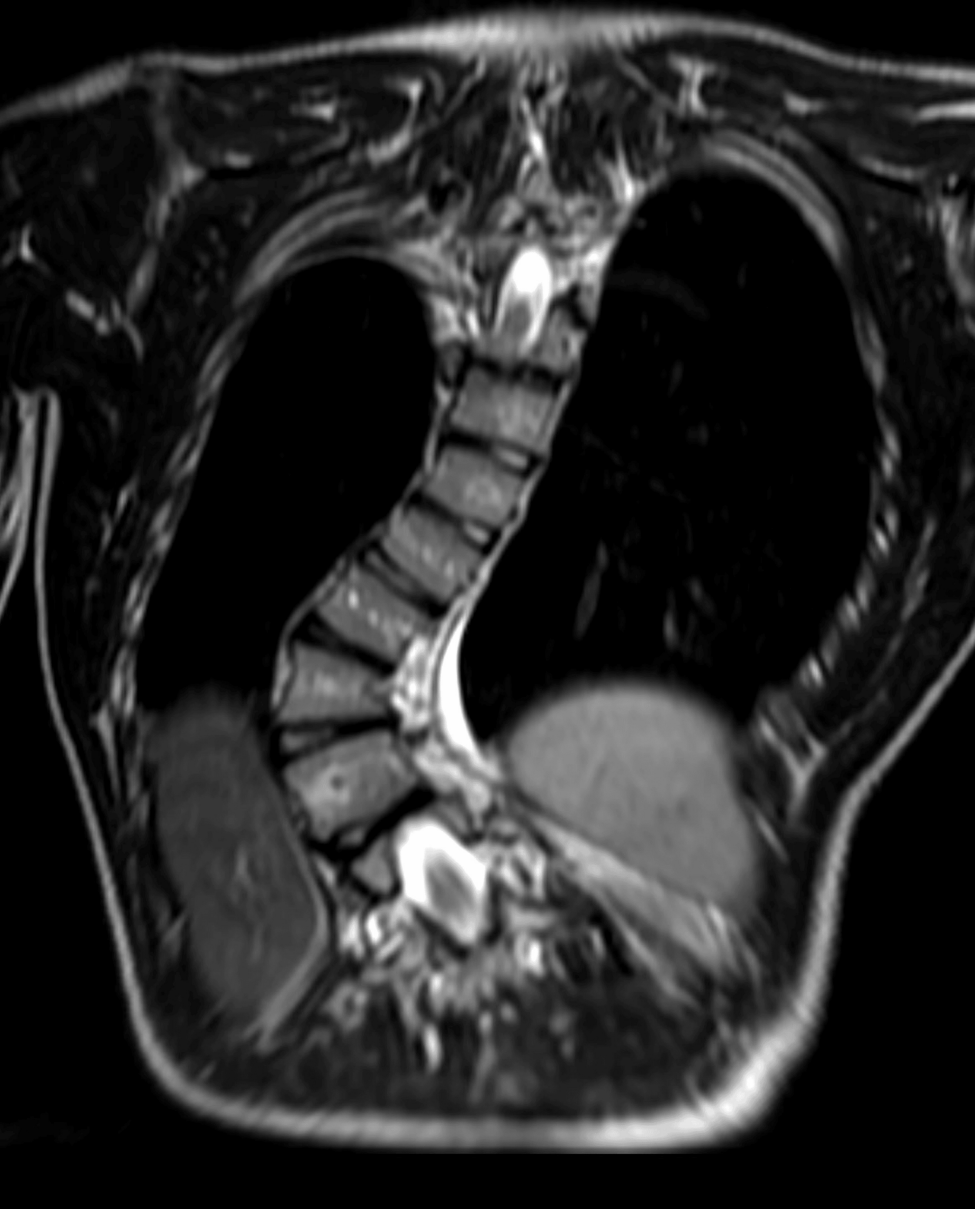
Coronal T2-weighted image of the thoracic spine showing dextrocurvature of the thoracic spine.
